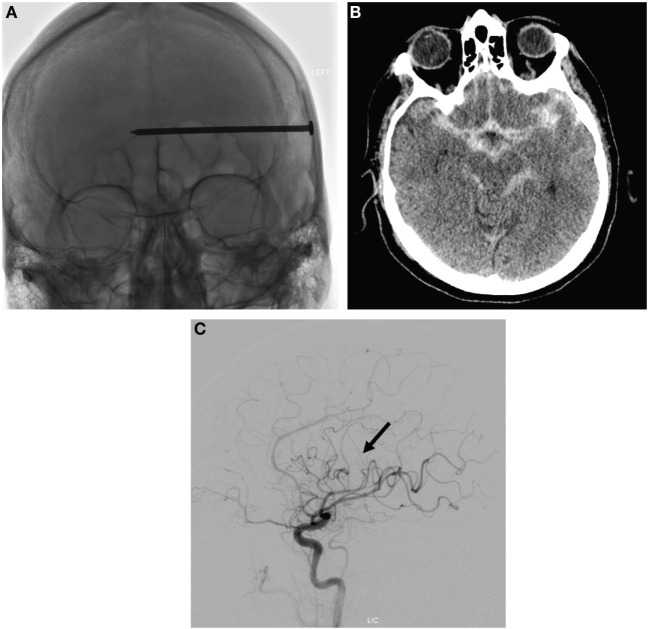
Assessment of initial injury from intracranial nail. (A) AP skull plain radiograph demonstrating nail penetration depth. (B) Axial image from head CT demonstrating basilar traumatic subarachnoid hemorrhage. (C) Lateral projection of a left internal carotid angiogram demonstrating nail positioning in the vicinity of a left M3 branch (nail head highlighted by black arrow), with no aneurysms, vessel occlusions/transections, or active extravasation.
